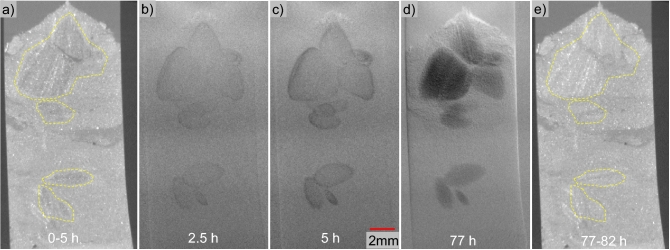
Overview CT images (a and e) and subtracted X-ray projections (b–d) showing salt diffusion in the mini-core. Note that (a) and (e) were an average of the mini-core during a period of 5 h, and (b–d) were the subtraction between the X-ray projections taken at the shown time and the X-ray projection taken at 0 h.
